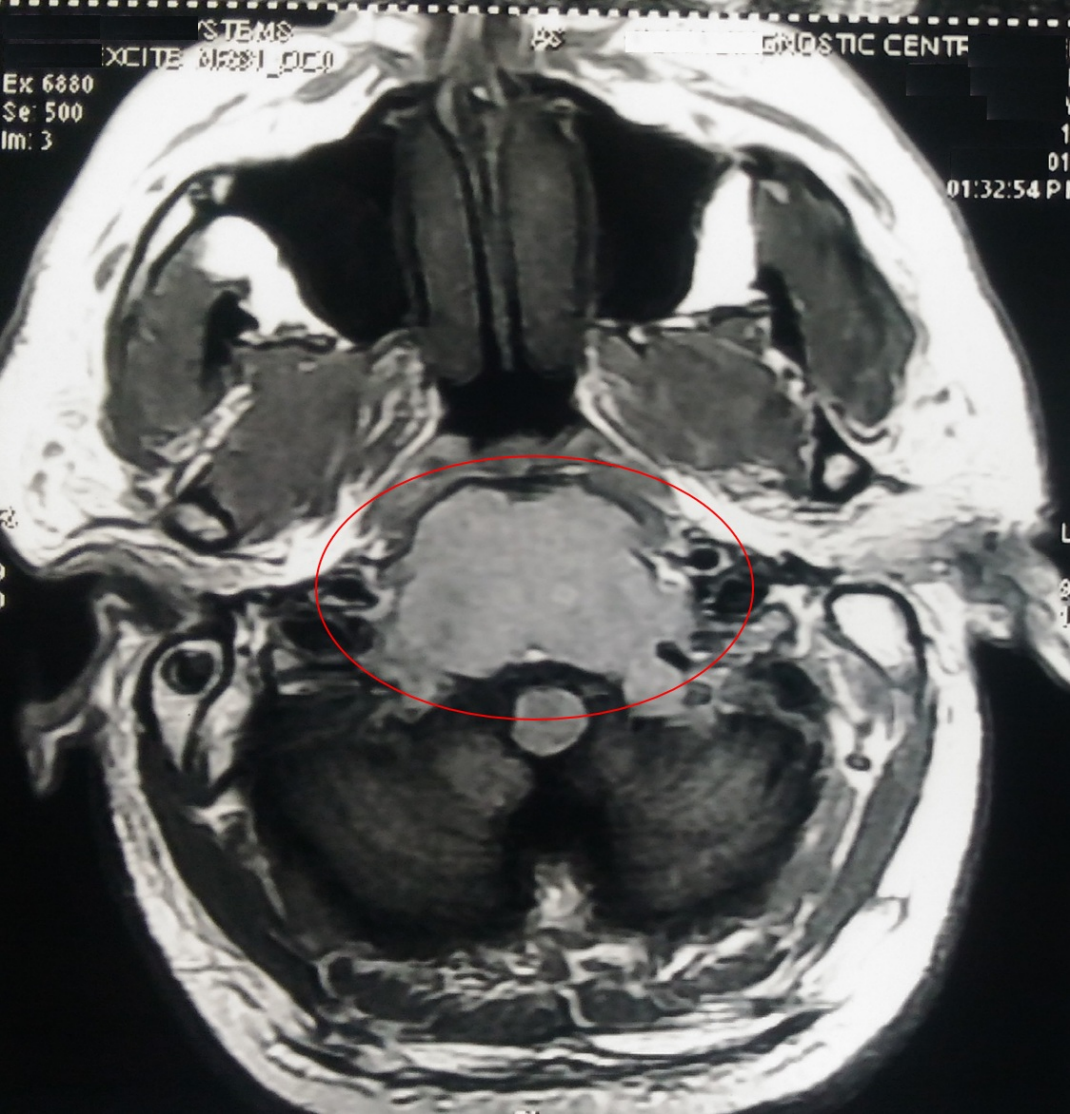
MRI showing a large heterogenous expansile lesion measuring 51 mm x 50 mm involving the central skull base, clivus with erosion of adjacent bones, and partially encasing bilateral ICA ICA: internal carotid artery; MRI: magnetic resonance image.
